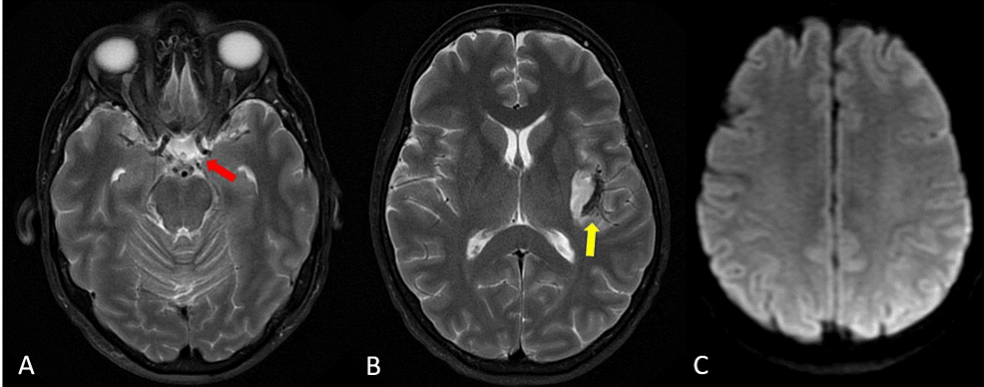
Follow-up Brain MRI Follow-up Brain MRI confirming small residual subarachnoid hemorrhage component within the left Sylvian fissure with no involvement of the basal cisterns (A, B). No procedure-related DWI+ findings within the left MCA posterior trunk vascular distribution (C). Yellow arrow indicating the SAH distribution. The red arrow was used as a reference to define where they would have been located the SAH in case of the left ICA aneurysm rupture.
